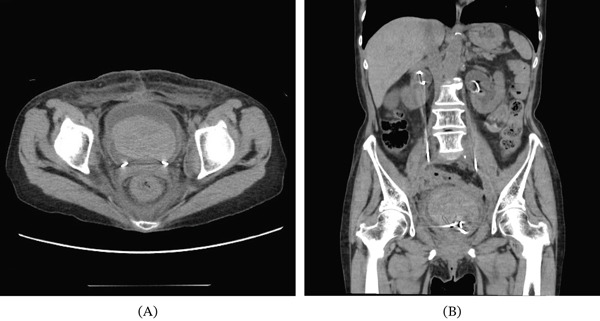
Noncontrast CT images on admission. (A) Intravesical hematoma. (B) No hematoma in the renal pelvis
